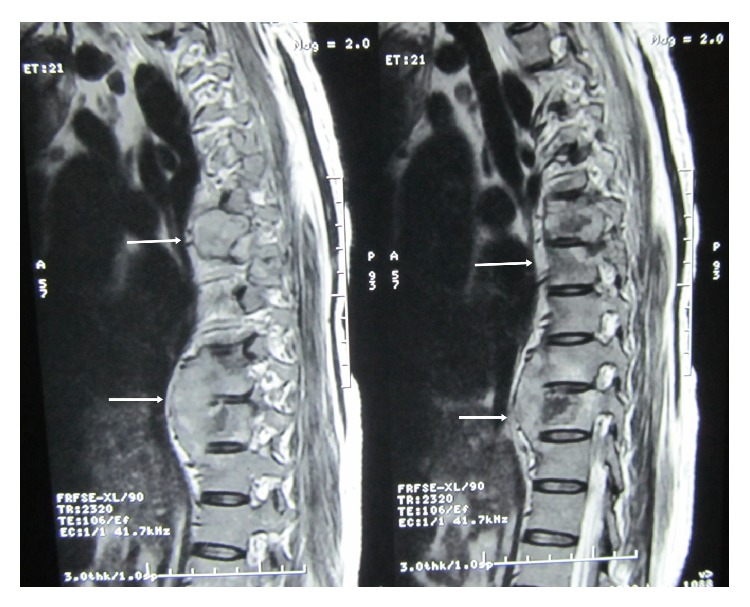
MRI of dorsal spine showing altered marrow signal intensity with abnormal soft tissue lesion involving D6, D7, D9, and D10 vertebrae and intraspinal extension with thecal sac compression at D6 vertebra.
